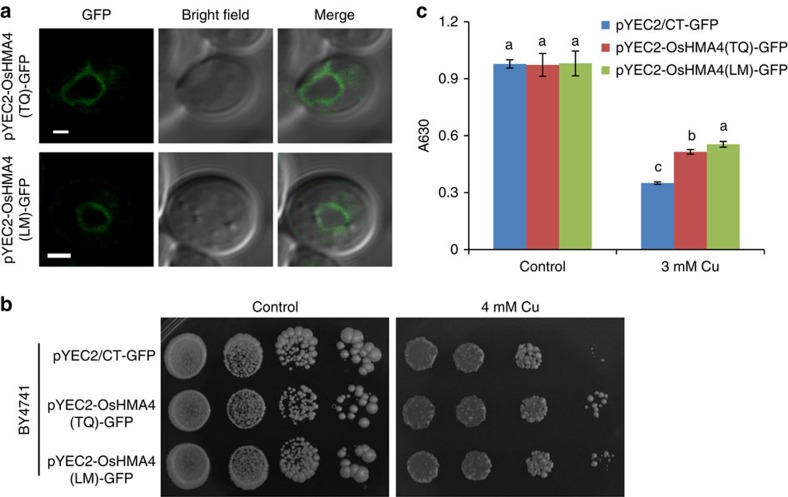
Functional analysis of OsHMA4 in yeast. (a) Subcellular localization of OsHMA4 in yeast. *OsHMA4* from TeQing (*OsHMA4(TQ)*) or from Lemont (*OsHMA4(LM)*) were expressed in a centromeric plasmid pYEC2/CT–GFP. GFP signals were observed mainly on the tonoplast. Bar, 1 μm. (b) Expression of *OsHMA4* in yeast using a centrimeric plasmid pYEC2/CT–GFP enhances Cu tolerance. Overnight yeast cell suspension of BY4741 transformed with empty vector pYEC2/CT–GFP or *OsHMA4* from TQ or LM were serially diluted (1:10) and spotted on the media without (Control) or with 4 mM CuSO_4_. Pictures were taken after 5 days growth at 30 °C. (c) The absorbance at 630 nm (A630) of cell cultures of BY4741 transformed with the empty vector pYEC2/CT–GFP or *OsHMA4-GFP* from TQ or LM. Yeast strains were grown in liquid media containing without (Control) or with 3 mM CuSO_4_ for 24 h. Data were shown as means±s.d. with three independent colonies. Columns with different italic or non-italic letters indicate significant difference under control or 3 mM CuSO_4_, respectively (*P*≤0.01, Fisher's LSD test).
